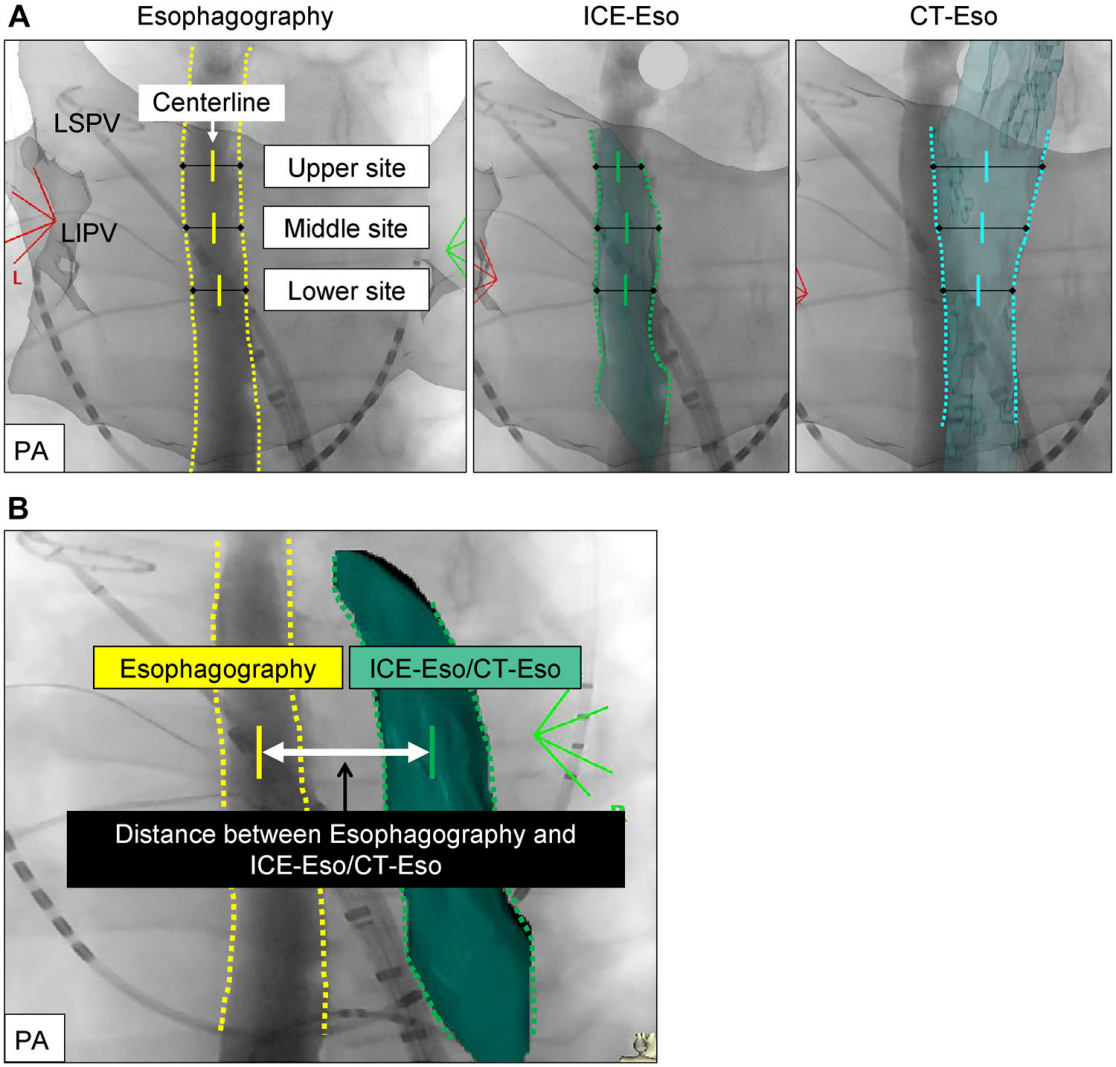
Comparison of Distance Between ICE‐Eso and Contrast Esophagography and Between CT‐Eso and Contrast Esophagography. Panel A: Definition of centerline in each imaging (*left panel*: Contrast esophagography; *middle panel*: ICE‐Eso; *right panel*: CT‐Eso). Panel B: Method of distance measurement between contrast esophagography and ICE‐Eso/CT‐Eso using the centerline of each image.
